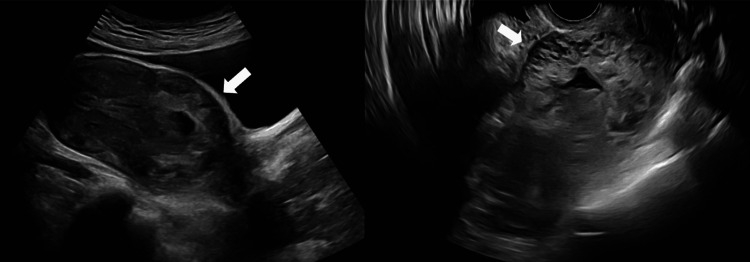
Transabdominal and transvaginal ultrasound images at the time of representation, indicating a low-lying gestational sac concerning for cesarean scar ectopic pregnancy. The arrow highlights the distinct uterine outpouching toward the bladder, which is pathognomonic of cesarean scar ectopic pregnancies. The hyperemic appearance around the gestational sac had significant vascularity, which was noted. These findings heightened suspicion for a partial molar pregnancy.
